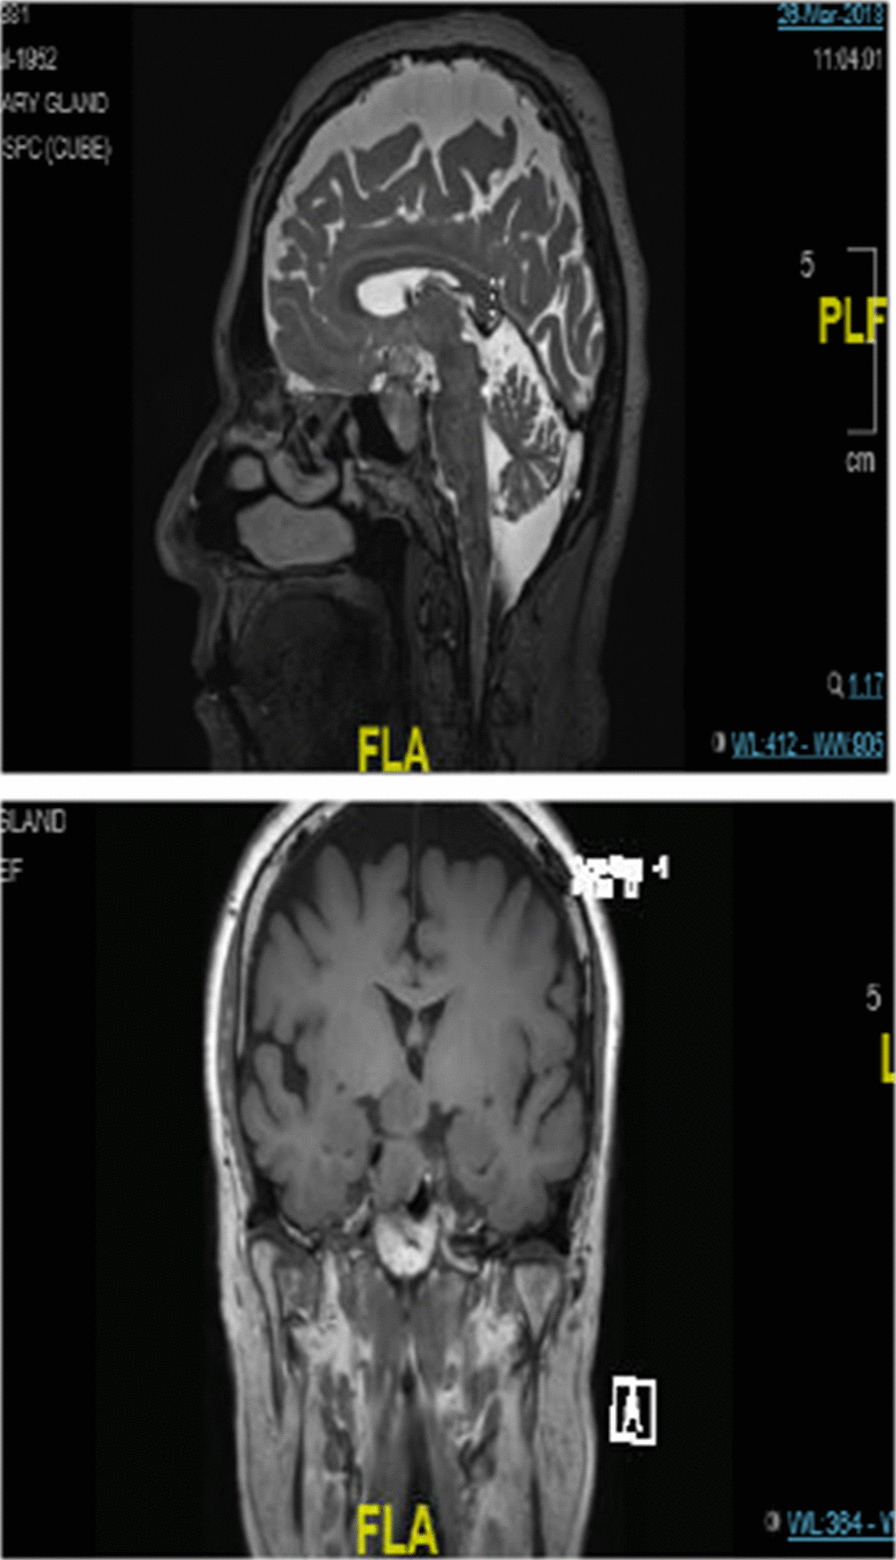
Baseline magnetic resonance imaging (MRI) pituitary showing a large sellar/suprasellar mass lesion causing compression and superior displacement on the optic chiasm as well as compression and lateral displacement of the neurohypophysis. Erosion of the roof of the sphenoid sinus with subsequent extension is also observed
